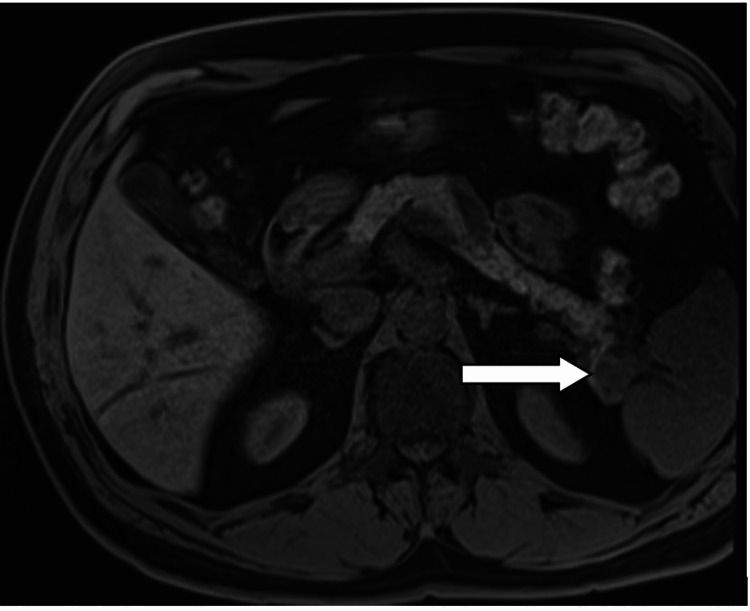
MRI abdomen transverse section revealing a 2.3 x 2.0 cm-sized lesion adjacent to the splenic hilum and tail of the pancreas
